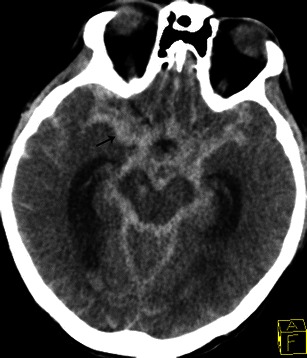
Non-contrast cerebral CT shows a spontaneous hyperdensity in the basal cistern due to subarachnoid haemorrhage (*black arrow*)
